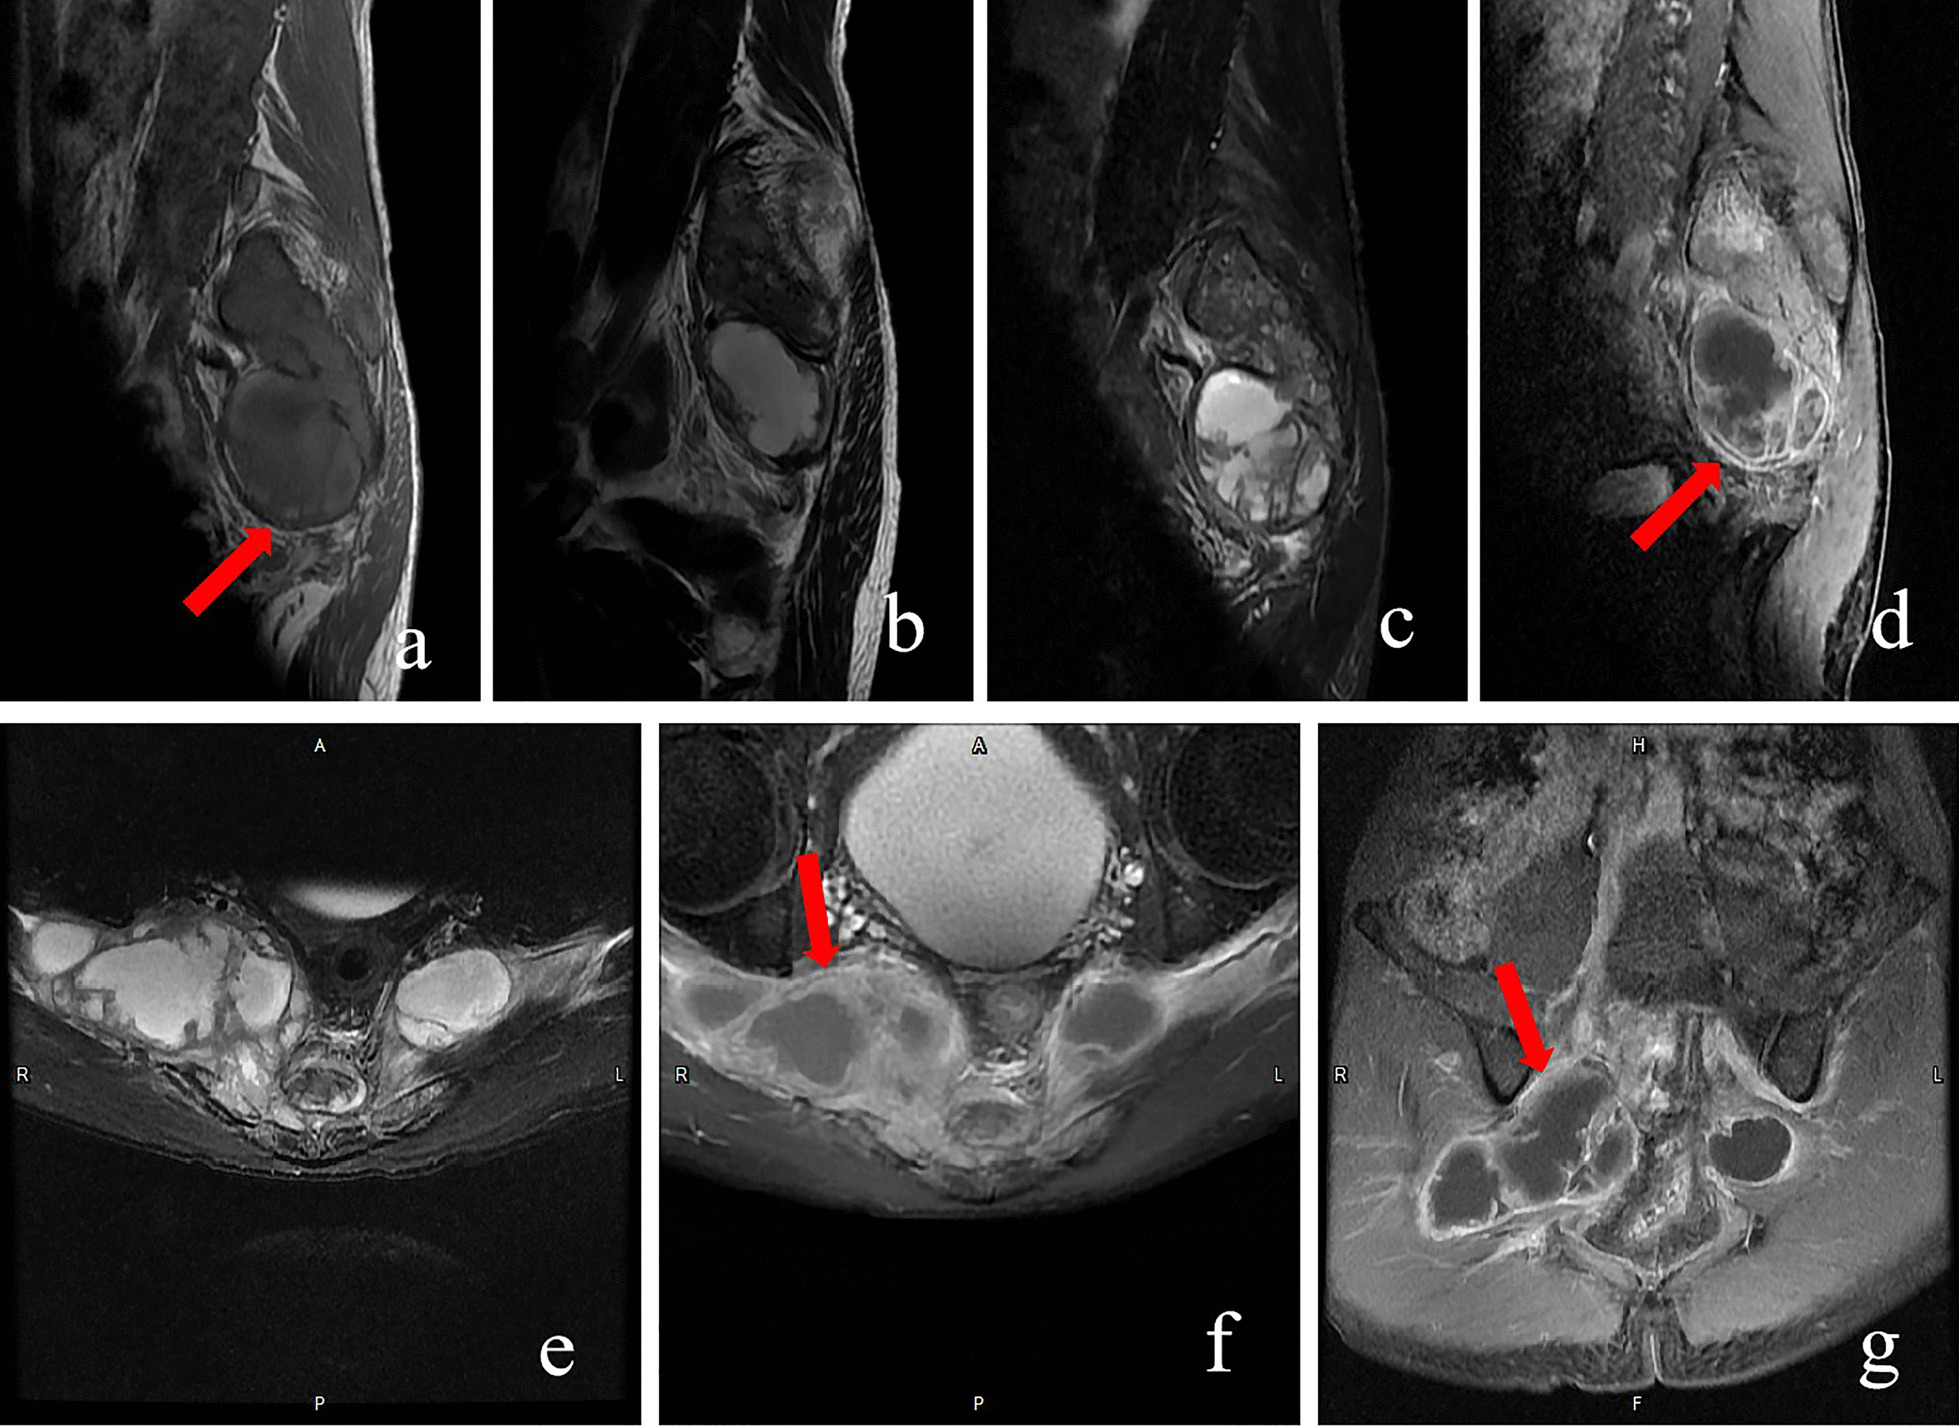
Preoperative magnetic resonance imaging (MRI) (sacrum). MRI (a sagittal T1-weighted; b sagittal T2-weighted; c sagittal fat-suppressed T2-weighted; e:transversal fat-suppressed T2-weighted): patchy abnormal signals in the sacrum and attached soft-cystic masses in the presacral, hyperintense signal on T2-weighted imaging (T2WI), and low-signal intensity on T1-weighted imaging (T1WI) (red arrows). Contrast-enhanced MRI (d sagittal enhanced T1-weighted; f transversal enhanced T2-weighted; g coronal enhanced T2-weighted): pre-sacral soft-tissue masses with thickened and enhanced soft cystic wall (red arrows)
